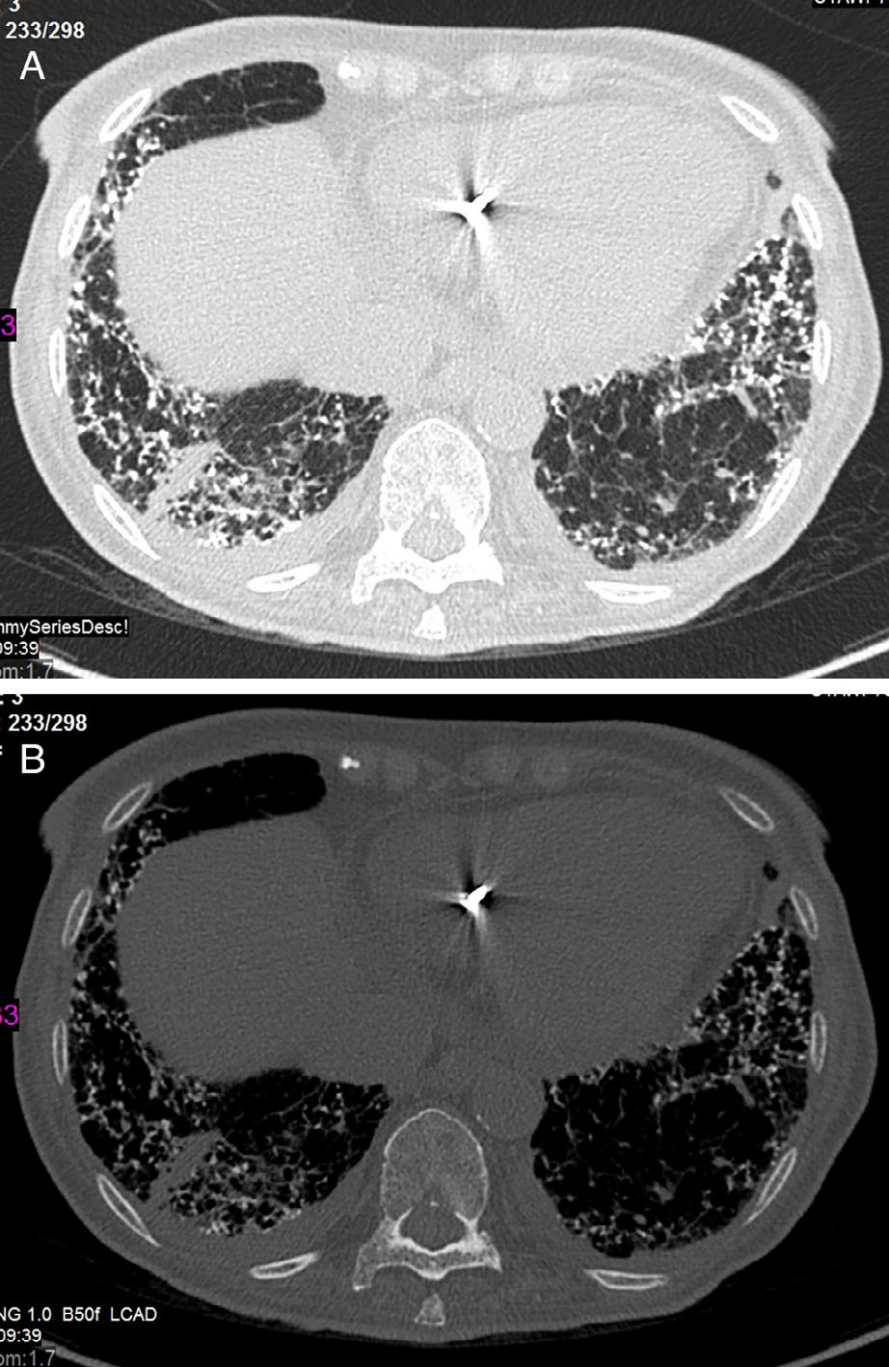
Case 3: A 93‐year‐old female with disseminated pulmonary ossification and chronic heart failure. (A) High‐resolution computed tomography shows nodular calcifications along the septa predominantly in the lower lobes. (B) On bone window setting, the calcifications are more conspicuous
